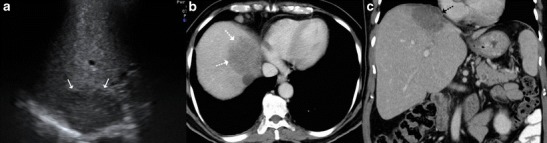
A 78-year-old man with weight loss and anorexia. a Ultrasound shows a well-defined hypoechoic lesion in the right lobe extending up to capsular surface (*arrows*). b, c Axial and coronal contrast-enhanced CT images show minimally enhancing subcapsular lesion in the right lobe (*white dashed arrow*) with associated capsular thickening (*black dashed arrow*)
